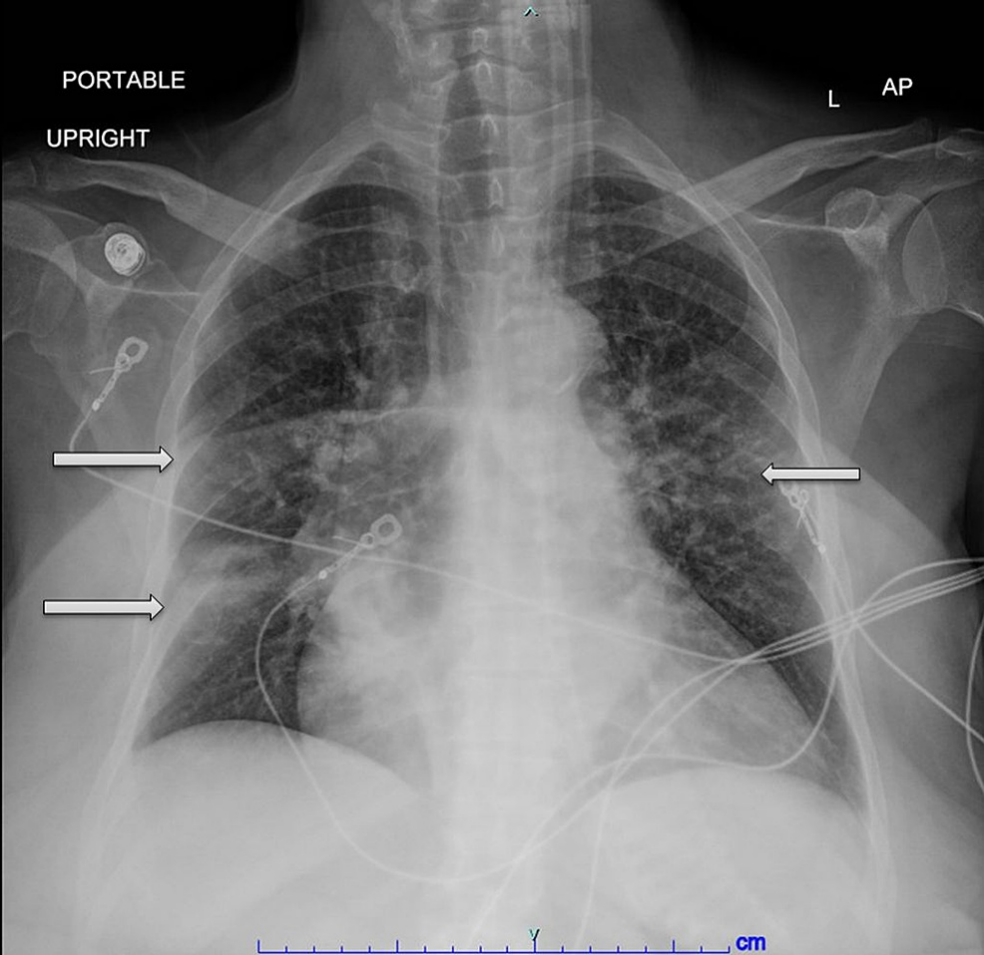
X-ray chest AP view; arrows show pulmonary opacities. AP, anteroposterior.
